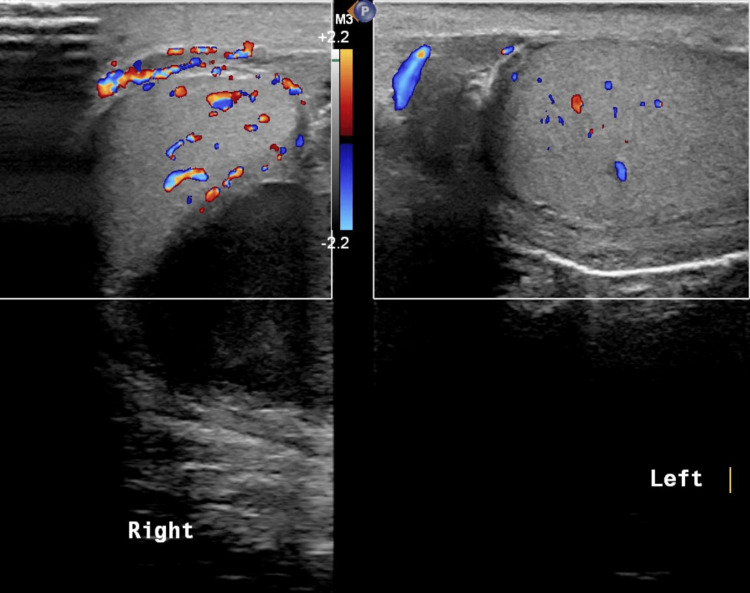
The scrotal ultrasound shows increased blood flow to the right testicle, with fluid collection around the right testicle.
